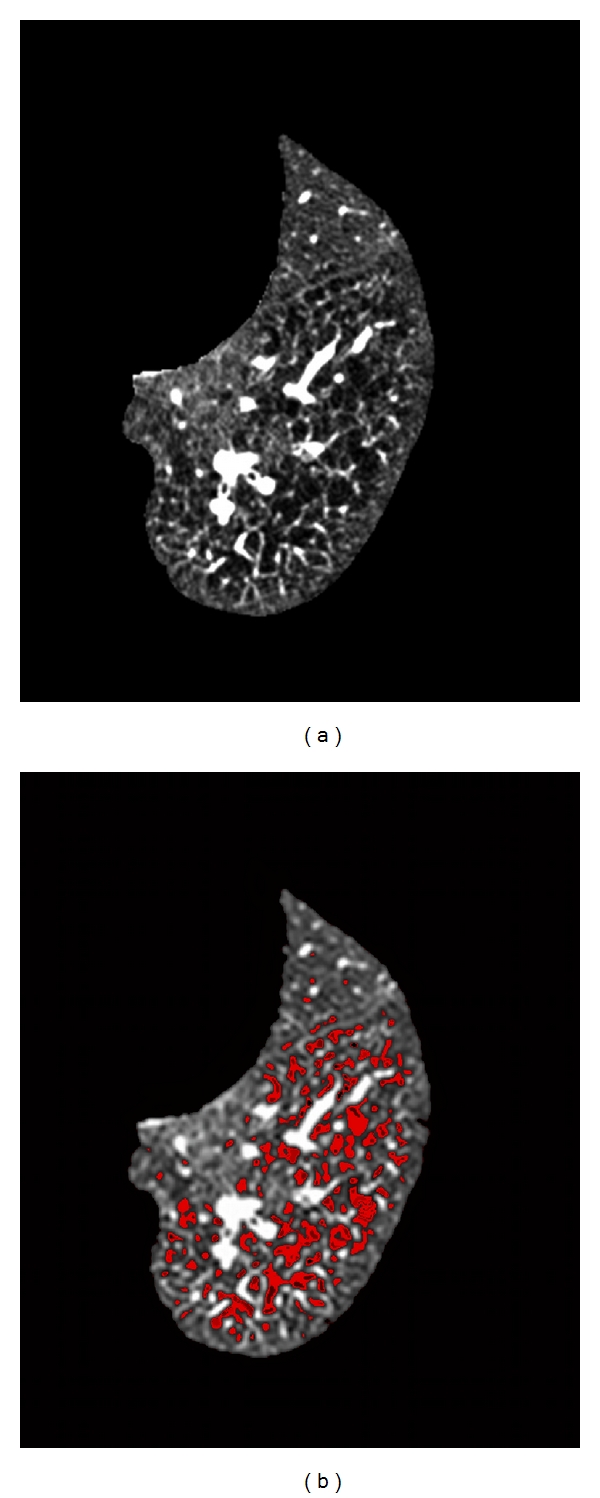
(a) Segmented thin-section CT image of basal left lung area in which lung contours and mediastinum were removed. (b) Same image using the density mask technique.
